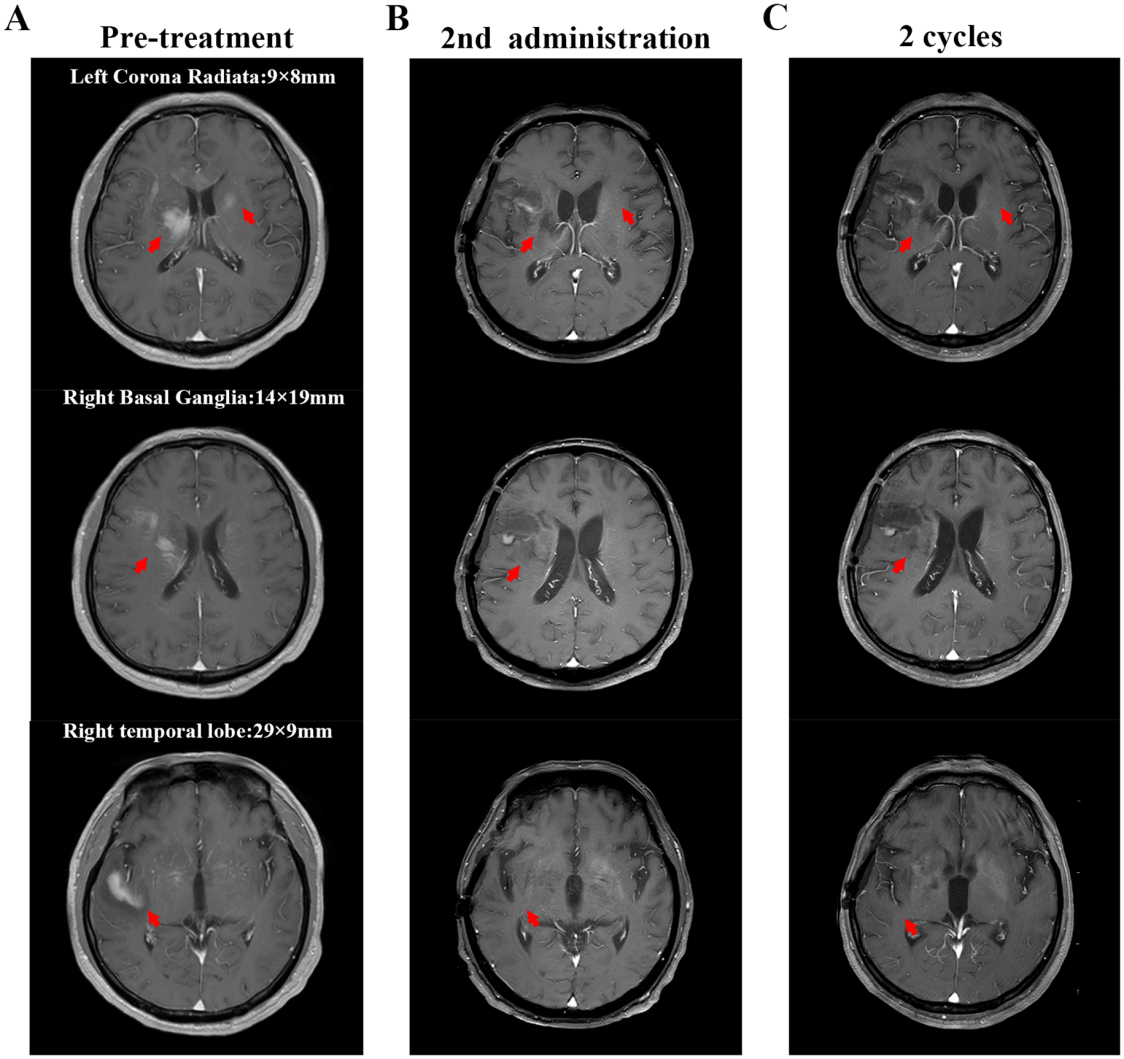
Cranial magnetic resonance imaging (MRI) findings. The red arrows indicate the intracranial lesion. (A) MRI scan shows the intracerebral lesions before treatment (including the right temporal lobe, right basal ganglia, and left corona radiata). The marked numbers indicate the size of the lesions; (B) MRI scan after the second administration of glofitamab; (C) MRI scan after two cycles of glofitamab administration. After treatment with glofitamab, the enhanced signal of the lesions on cranial MRI decreased, making it impossible to accurately measure their sizes.
